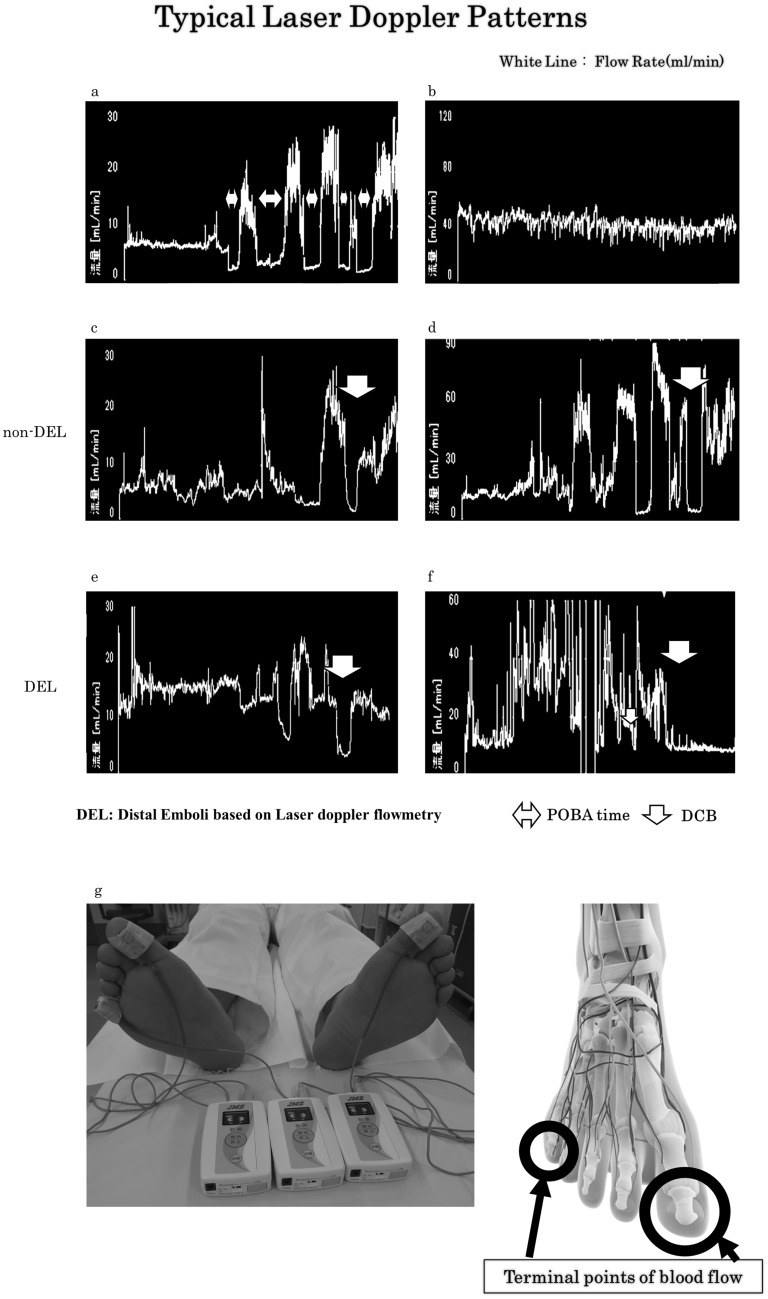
Graphs show typical laser doppler patterns during balloon angioplasty. White line shows flow rate (mL/min). a, c, d Typical improved cases. A drop in POBA time is seen (double arrows). Soon after POBA, flow rate increased soon after POBA. b Control data patterns. Flow rates are unchanged or stable. e, f Typical distal embolism patterns. There are two main patterns: one in which the flow drops gradually (e) and one in which the flow drops suddenly (f). g Attaching the laser doppler device. We chose the first and fifth toes as the terminal points of blood flow. We used the first and fifth toes of the contralateral leg as controls. *POBA* plain old balloon angioplasty
